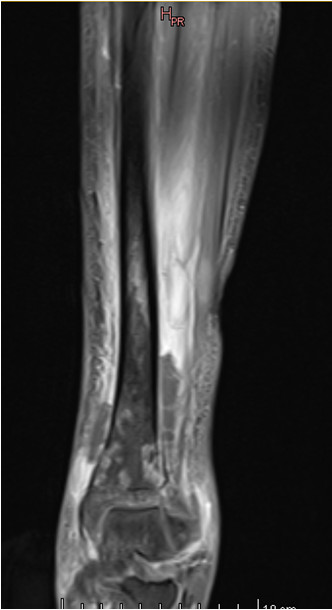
Initial magnetic resonance images of the affected tibia. Overall extension is best understood on magnetic resonance images with extensive heterogeneous medullar and pericortical enhancement on T1-weighted fat-suppressed coronal images.
